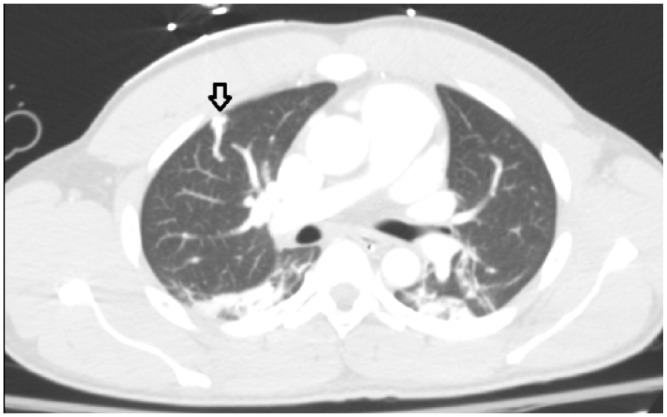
CT chest with contrast shows a focus within the inferior right upper lobe with a prominent feeding artery 2.9 mm in diameter, consistent with an arteriovenous malformation.
